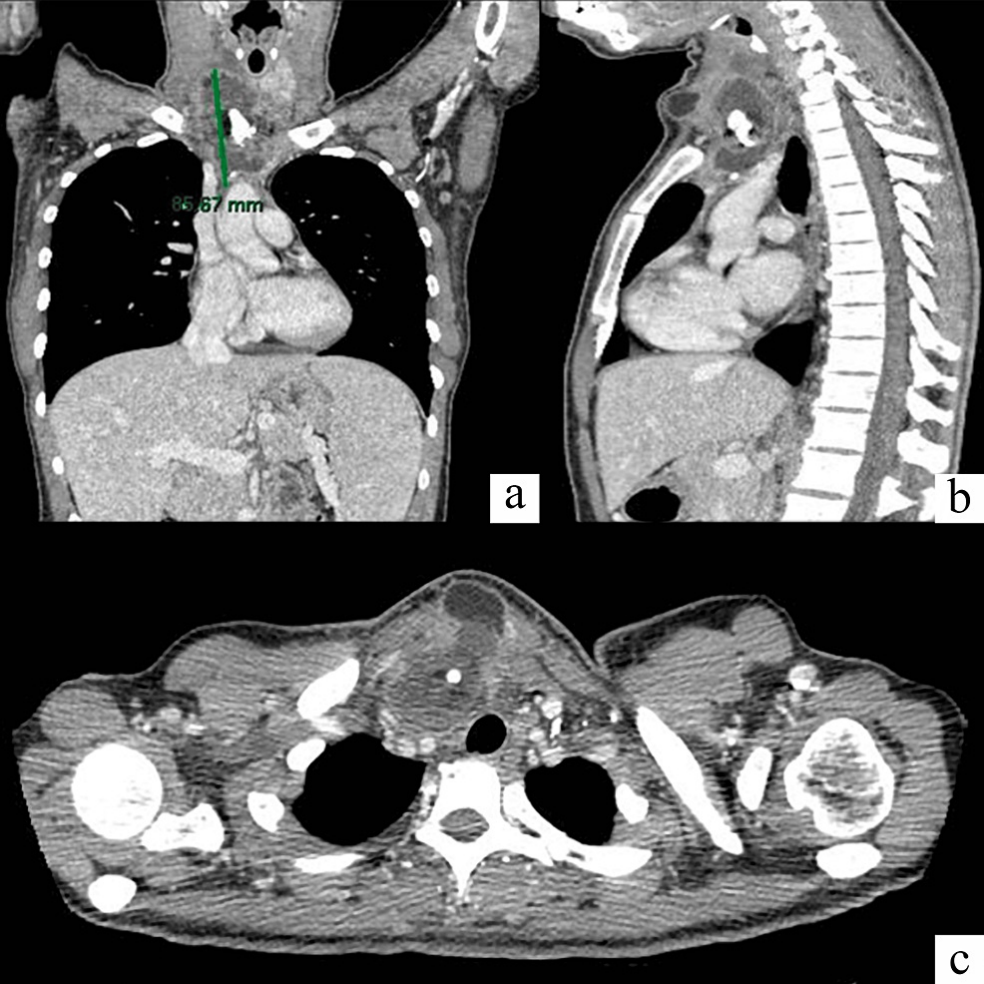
Neck-chest CT scan a) Coronal; b) Sagittal; c) Axial CT scan
